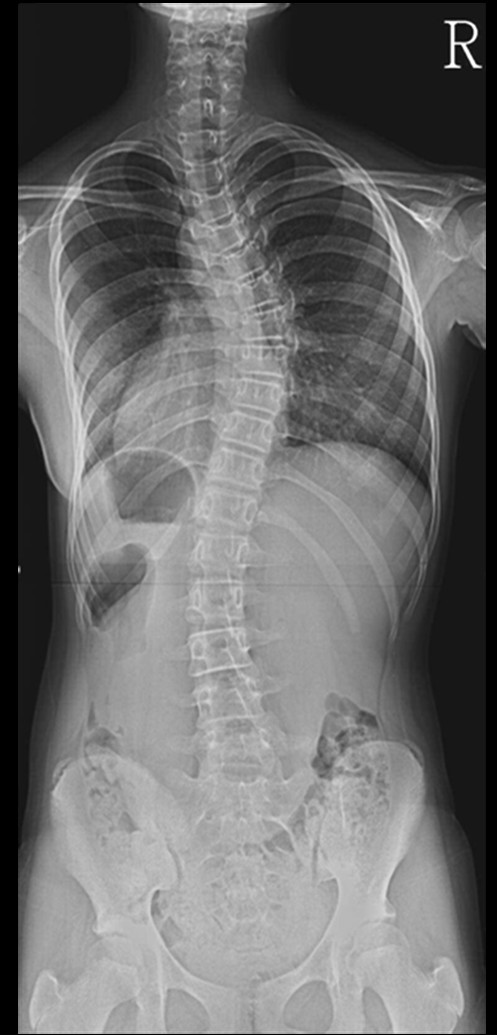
Roentgen graphic assessment was performed with whole spine standing radiogram including pelvis in all patients.
